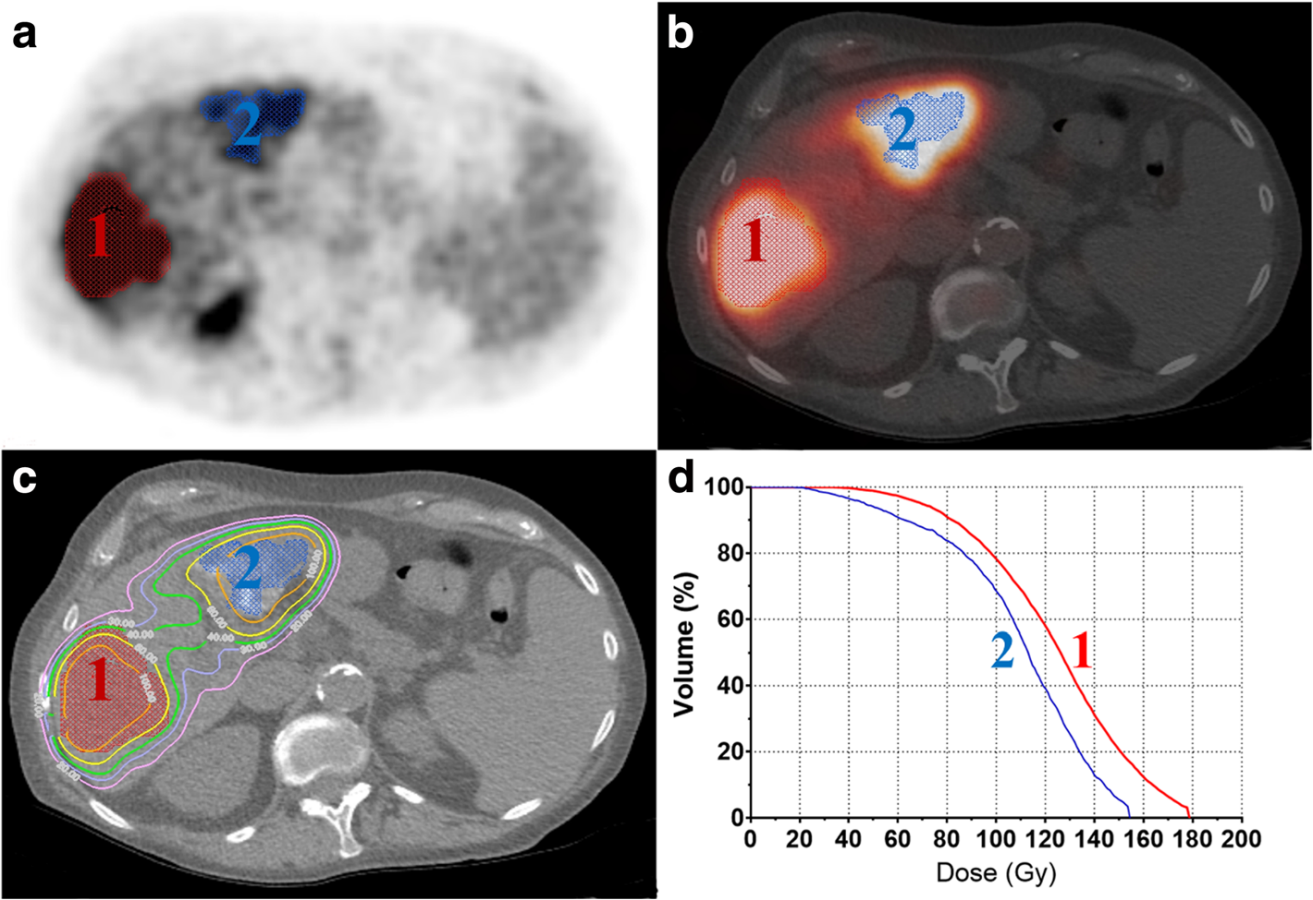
Post-treatment dosimetry process. a Baseline FDG-PET shows the delineated lesions (1 and 2). b Axial ^90^Y-PET/CT image on which two target lesion volumes have been projected, showing a high ^90^Y-microsphere uptake. c
